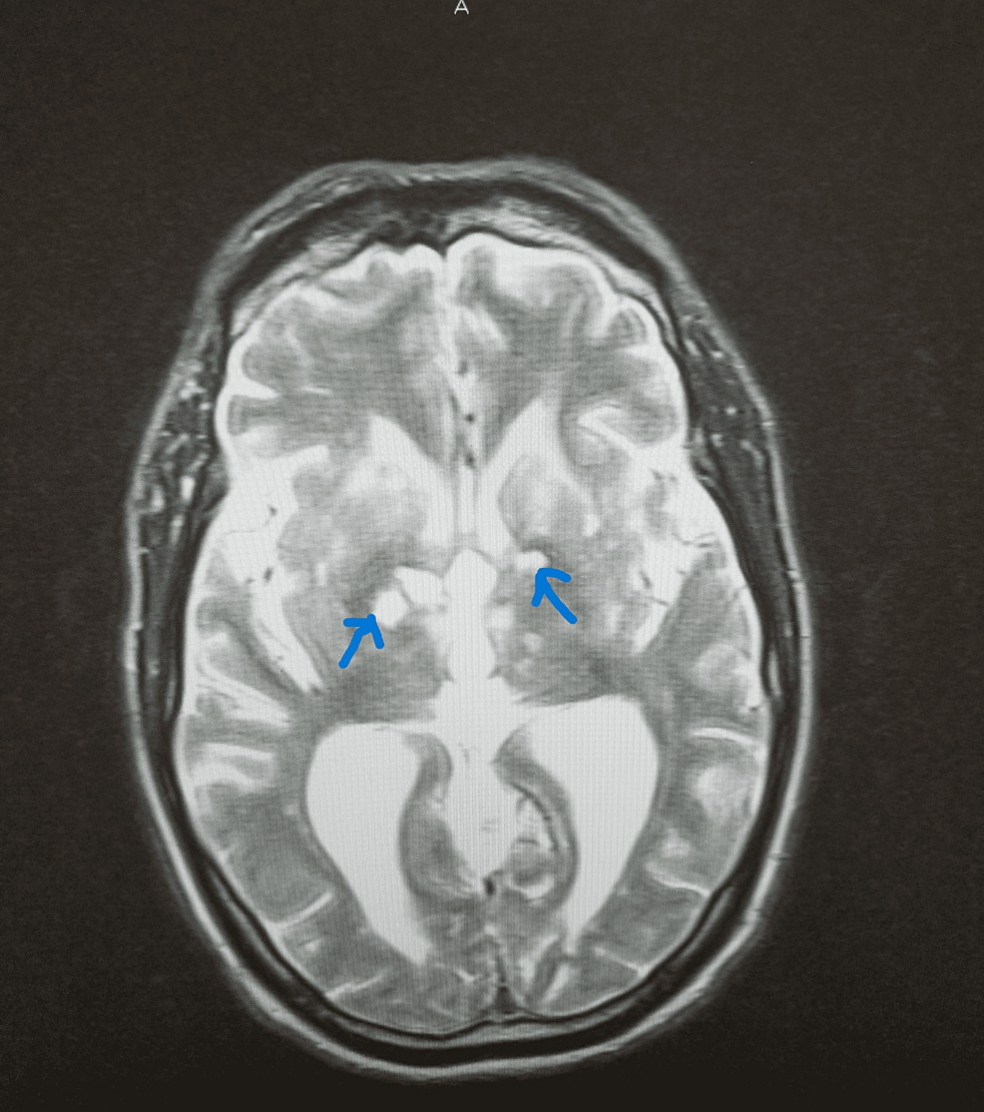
MRI brain w/stem without contrast showing bilateral thalamic and basal ganglia infarcts.
